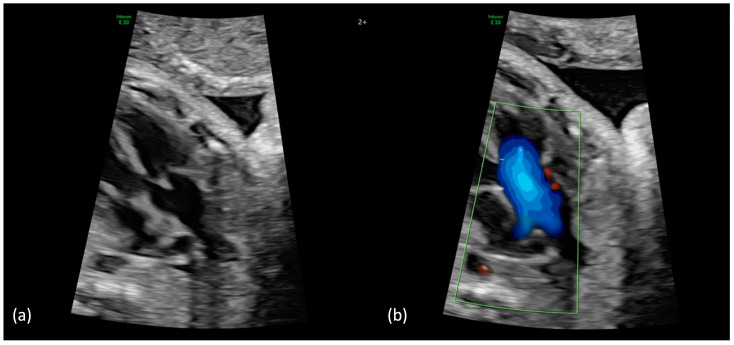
(a,b): Truncus arteriosus type 1 at 31 + 4 weeks. (a) Perimembranous outlet VSD as interventricular communication with the broad common arterial trunk in the overriding position. (b) In the color Doppler mode, the filling of the common arterial trunk from both ventricles and the posterior branch of one pulmonary artery can be seen (aortic dominance); aliasing or a high variance, which indicates a stenosis of the truncal valve, is not present.
